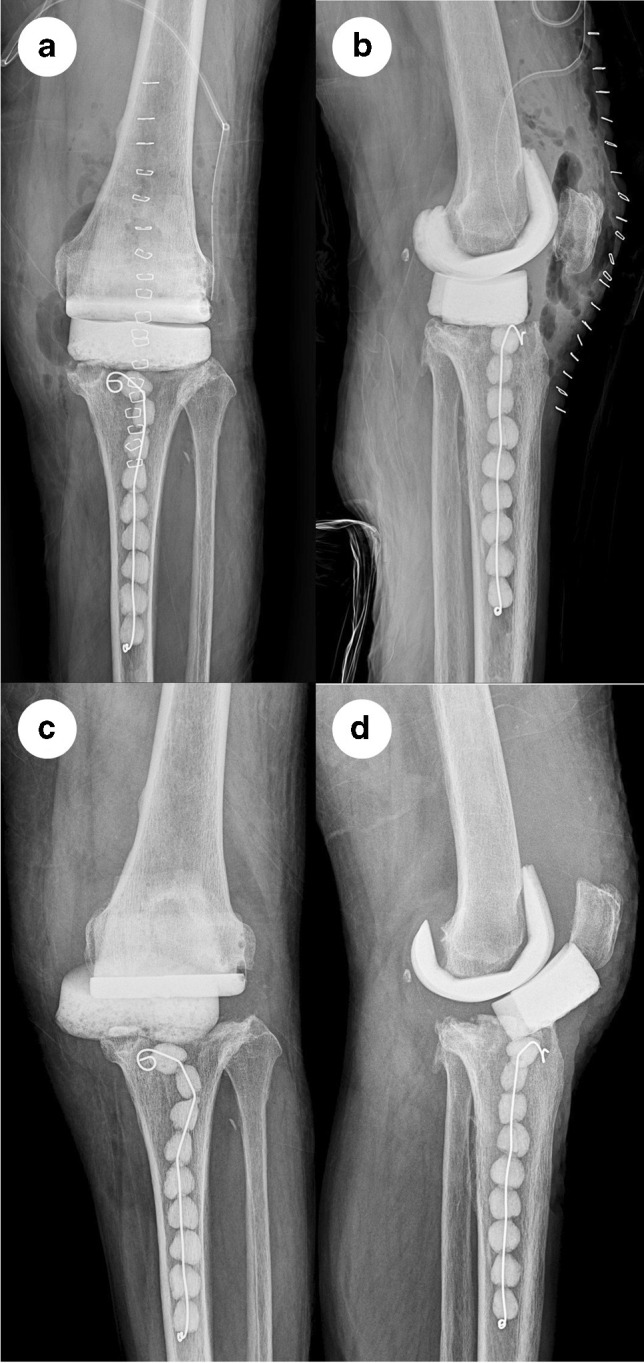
Anteroposterior (a) and lateral (b) radiographs of a 76-year-old woman after the first stage of revision total knee arthroplasty (TKA). Radiographs (c, d) prior to the second stage of revision TKA showed that the tibial cement spacer translated medially and tilted more
